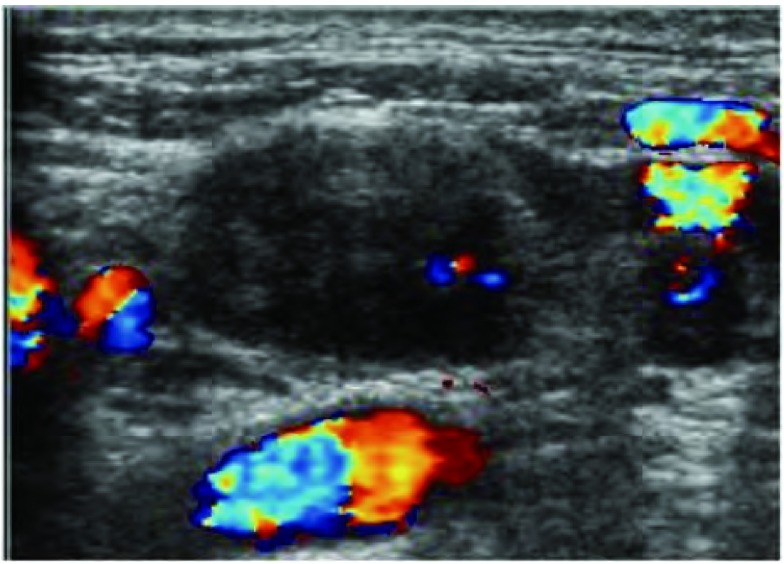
锁骨上转移性淋巴结在声像图中表现为低回声结节，皮髓质分界不清，内部及周边可见血流信号。 The ultrasound image performance of supraclavicular metastasis lymph nodes were hypoechoic nodules with cortex and medulla unclear boundaries and internal and peripheral blood flow signals.
